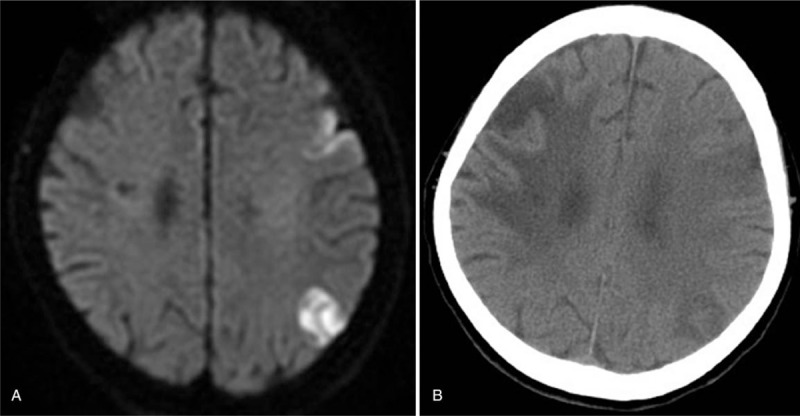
(A) Diffusion-weighted image. Hyperintense areas are observed in the left cortex and the posterior part of the middle cerebral arterial territory. (B) Computed tomography showing left cerebral infarction without hemorrhagic changes in the same site.
